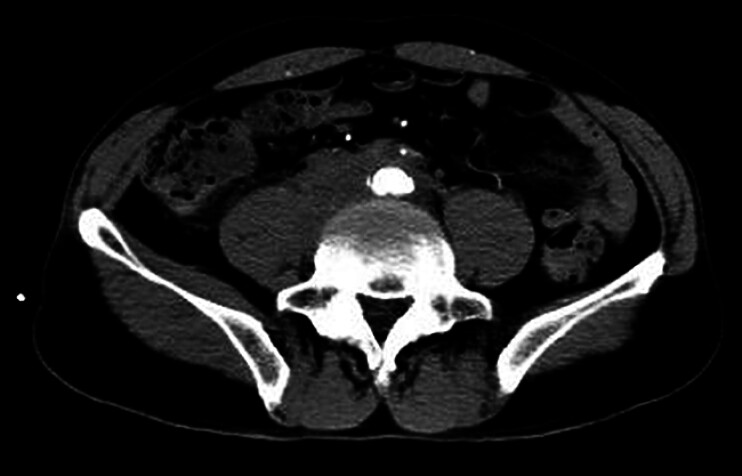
Contrast-enhanced computed tomography showed an abnormal retroperitoneal soft tissue mass encircling the abdominal aorta.
